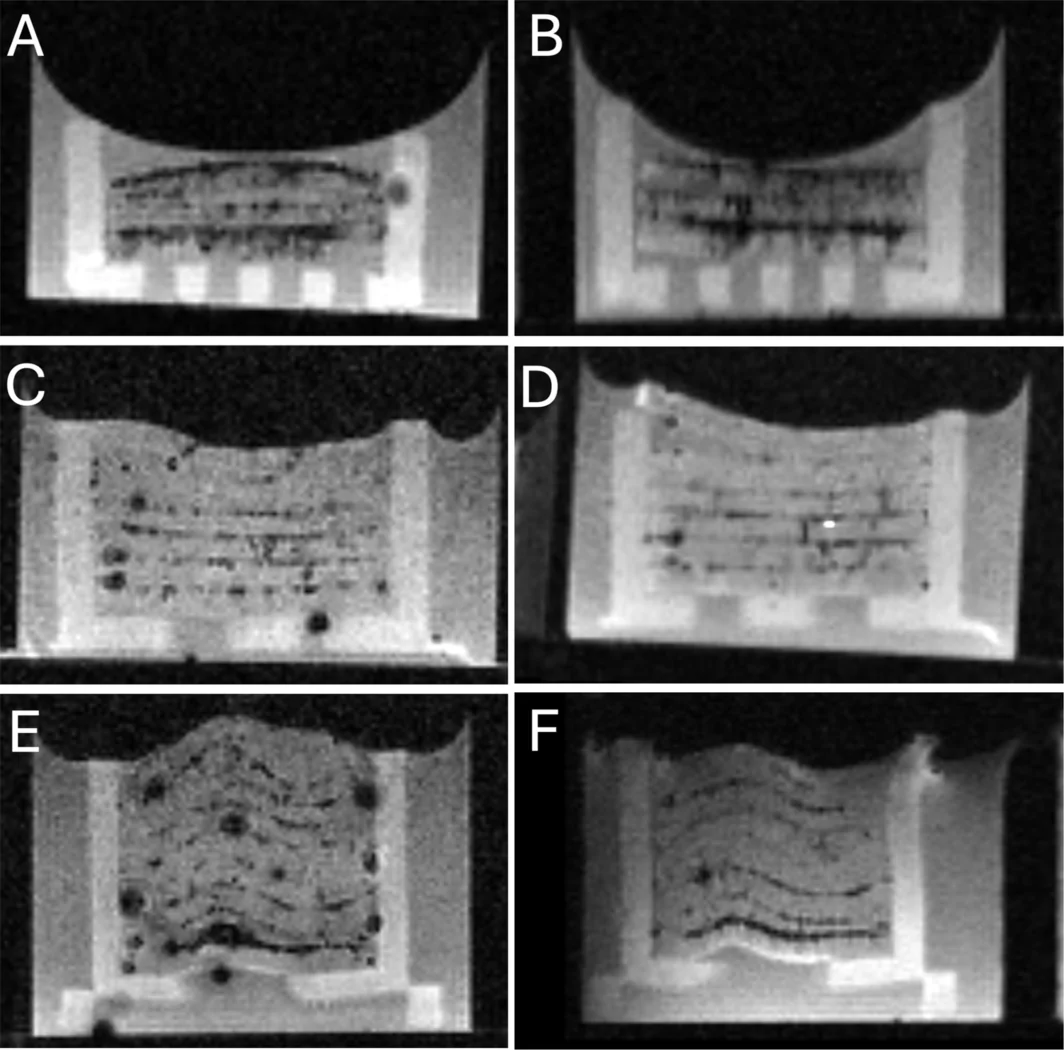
Longitudinal MRI assessment of multilayer biopaper constructs containing labelled cells. (A,B) MR images of the three‐layer construct acquired on Day 1 (A) and Day 28 (B). (C,D) MR images of the six‐layer construct acquired on Day 1 (C) and Day 28 (D). (E,F) MR images of nine‐layer constructs acquired on Day 1 (E) and Day 28 (F).
